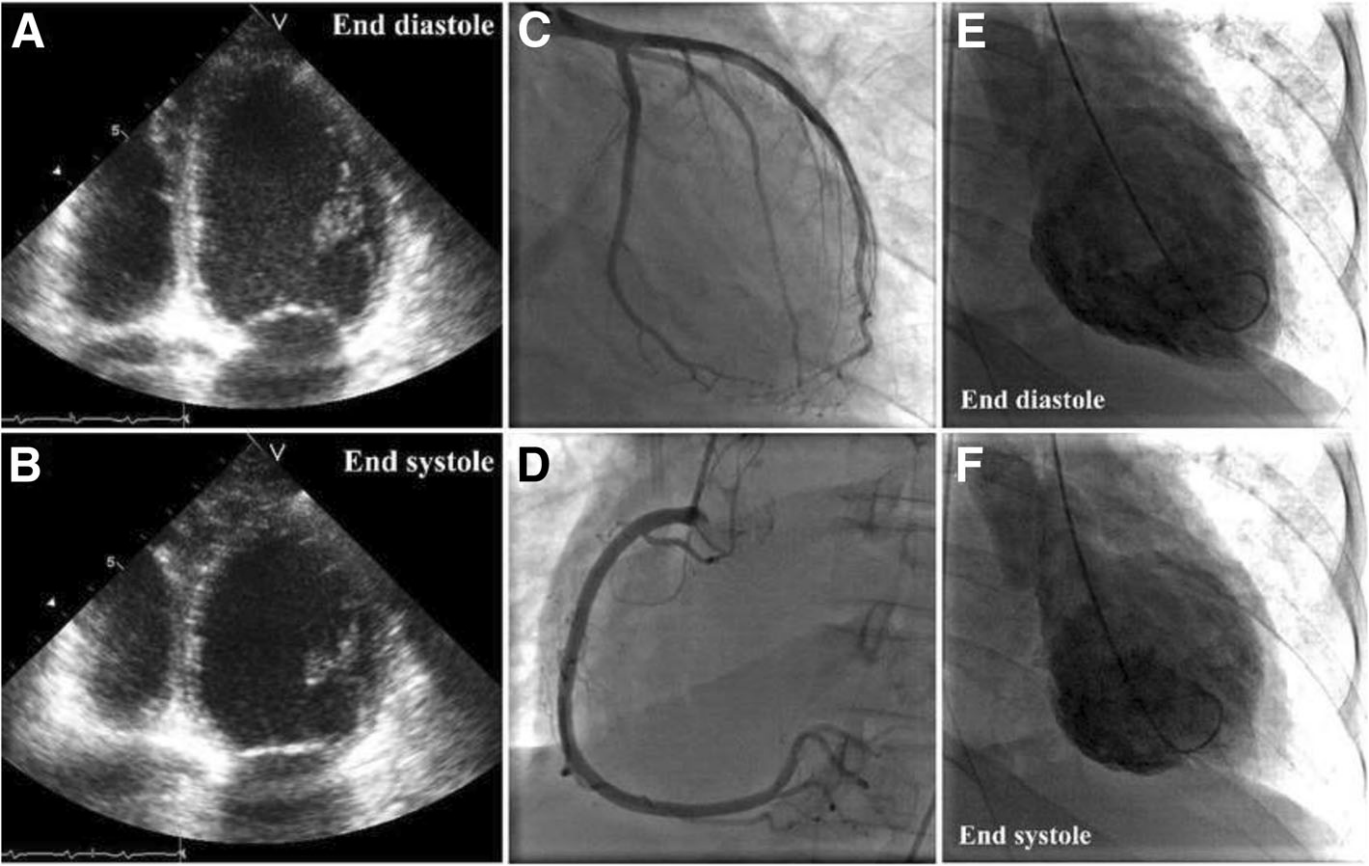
Echocardiographic four-chamber view (a, b), coronary angiography (c, d), and ventriculography (e, f) of the patient during the acute phase
